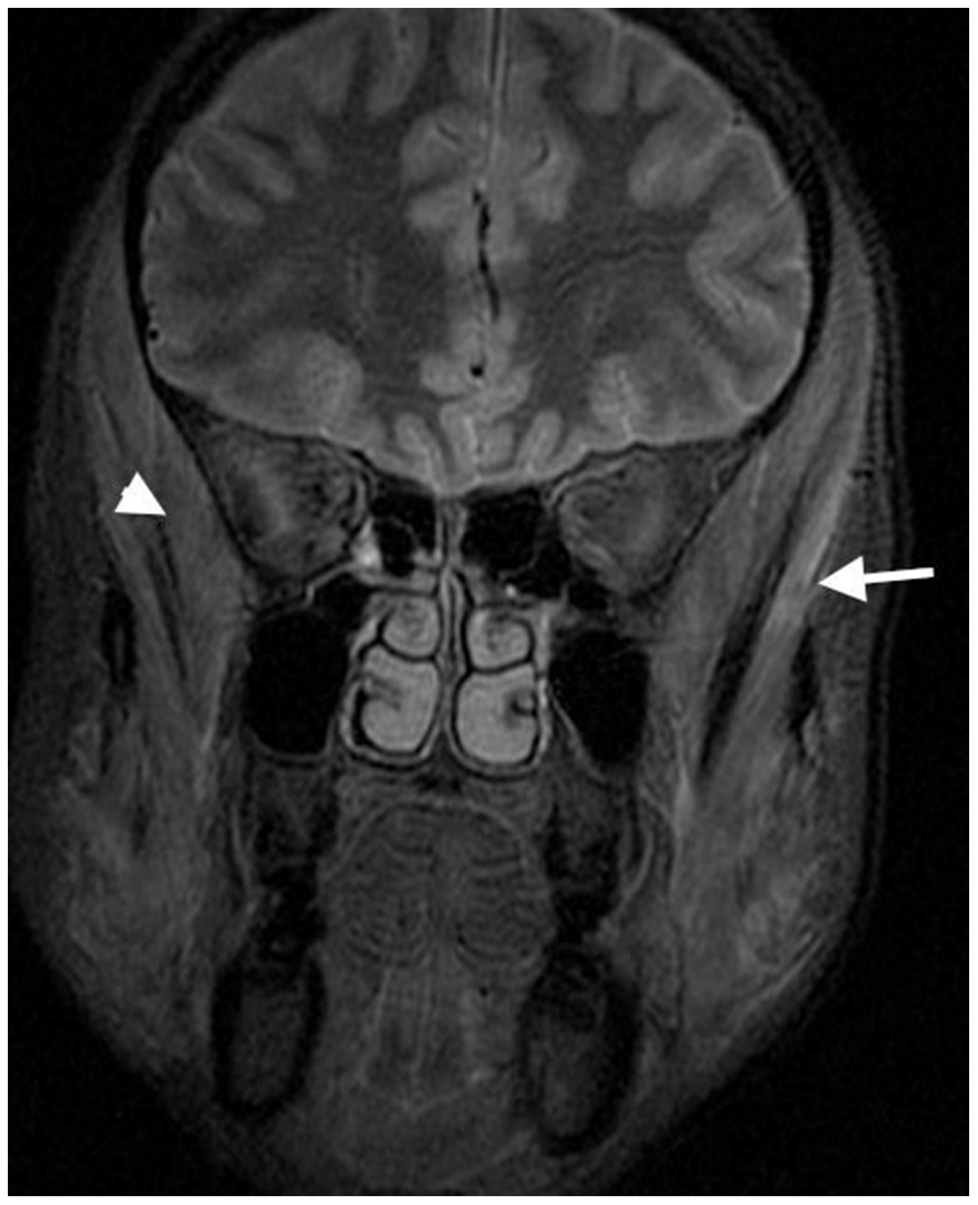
Coronal STIR MRI demonstrating bilateral asymmetrical high signal in deep (arrow head) and superficial (arrow) temporalis muscles (Case 1, Table 3).
